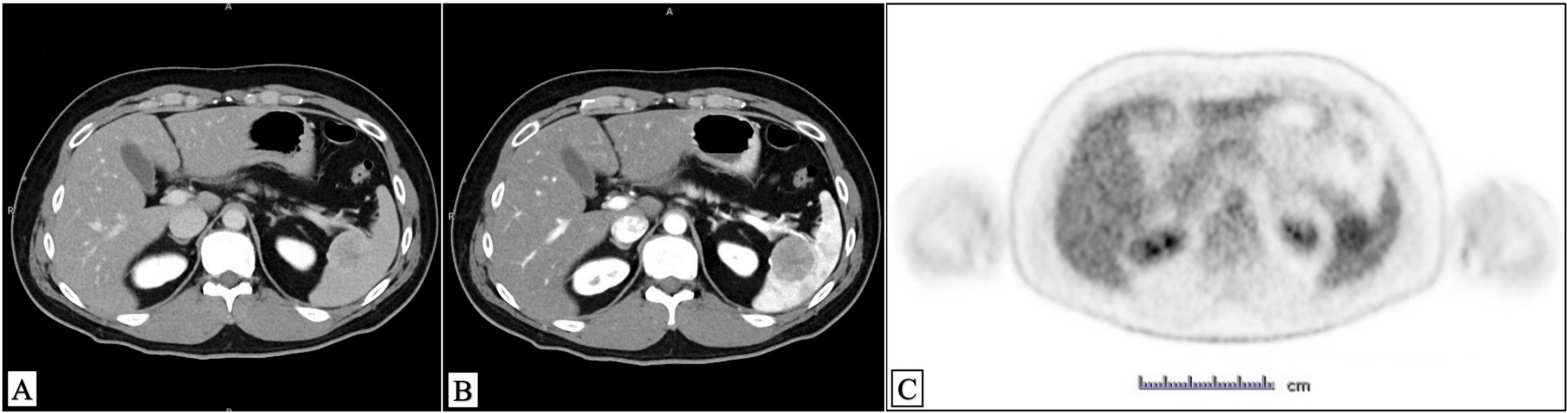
Radiological imaging (A, B) Contrast-enhanced computed tomography (CT) revealed a hypodense lesion in the spleen with relatively well-defined borders, measuring approximately 3 cm in diameter. In the early phase (A), the lesion showed low attenuation, while in the delayed phase (B), it demonstrated continuous internal arborizing enhancement. (C) Positron emission tomography (PET) demonstrated a solitary splenic mass measuring approximately 3 cm in diameter with a moderately increased fluorodeoxyglucose (FDG) uptake, heterogeneous in nature, and no evidence of distant metastasis.
